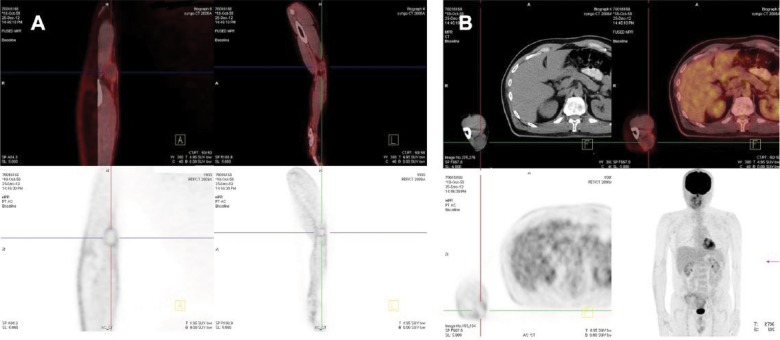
Follow-up FDG PET scan (axial, coronal, sagittal views): (a) There is moderate uptake of tracer in the surgical area of the right epitrochlear region and (b) normal uptake of FDG in the whole body.
